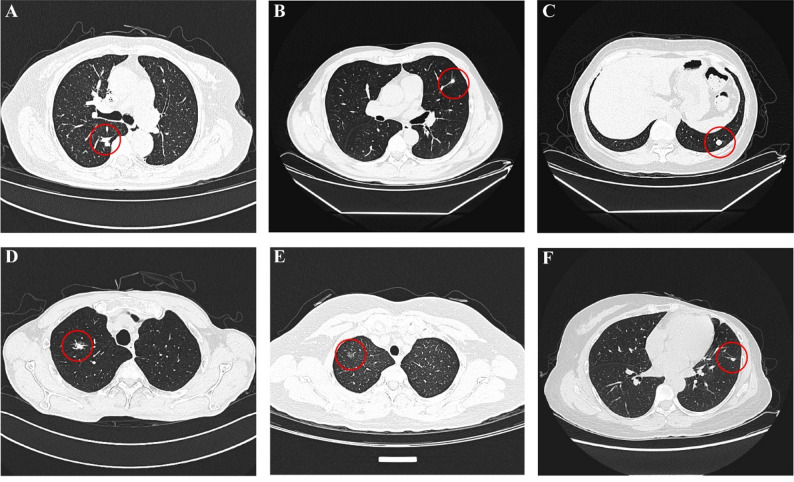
Representative CT images of pulmonary nodules demonstrating key imaging features. Case A: Patient in their 70s with chronic granulomatous inflammation, showing a nodule in the posterior segment of the right upper lobe with irregular morphology, lobulated margins, and pleural indentation sign, demonstrating imaging features mimicking malignancy. Case B: Patient in their 40s with an inflammatory lesion, displaying a solid nodule measuring approximately 7 mm × 6 mm in the superior lingular segment of the left upper lobe with lobulation, spiculated margins, and vascular convergence sign, also showing imaging characteristics suggestive of malignancy. Case C: Patient in their 50s with hamartoma, showing a round nodule with smooth margins and no internal calcification in the posterior basal segment of the left lower lobe, demonstrating typical benign imaging features. Case D: Patient in their 60s with invasive pulmonary adenocarcinoma, demonstrating a part-solid (mixed ground-glass) nodule in the apical segment of the right upper lobe with irregular morphology, spiculated margins, and solid component exceeding 5 mm, showing definite imaging features of malignancy. Case E: Patient in their 60s with minimally invasive adenocarcinoma, presenting a ground-glass nodule in the apical segment of the right upper lobe with ill-defined margins, measuring approximately 9 mm in maximum diameter, with small vessels penetrating into the lesion, demonstrating features consistent with malignancy. Case F: Patient in their 40s with metastatic carcinoma and known history of breast cancer, showing a small nodule adjacent to the oblique fissure pleura in the left lower lobe with well-defined margins, closely abutting the interlobar pleura, without lobulation or spiculation; despite benign imaging appearance, metastasis cannot be excluded given the patient’s oncologic history
